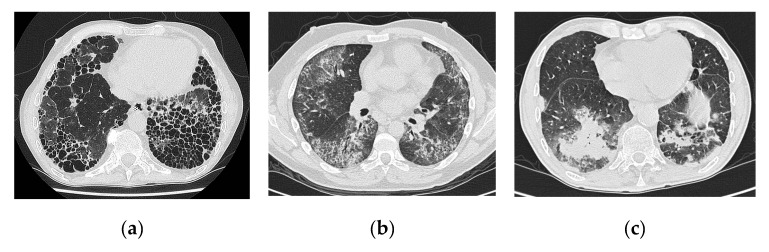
HRCT images showing (a) rheumatoid arthritis-associated usual interstitial pneumonia (RA-UIP), (b) rheumatoid arthritis-associated non-specific interstitial pneumonia (RA-NSIP), and (c) rheumatoid arthritis-associated organizing pneumonia (RA-OP).
